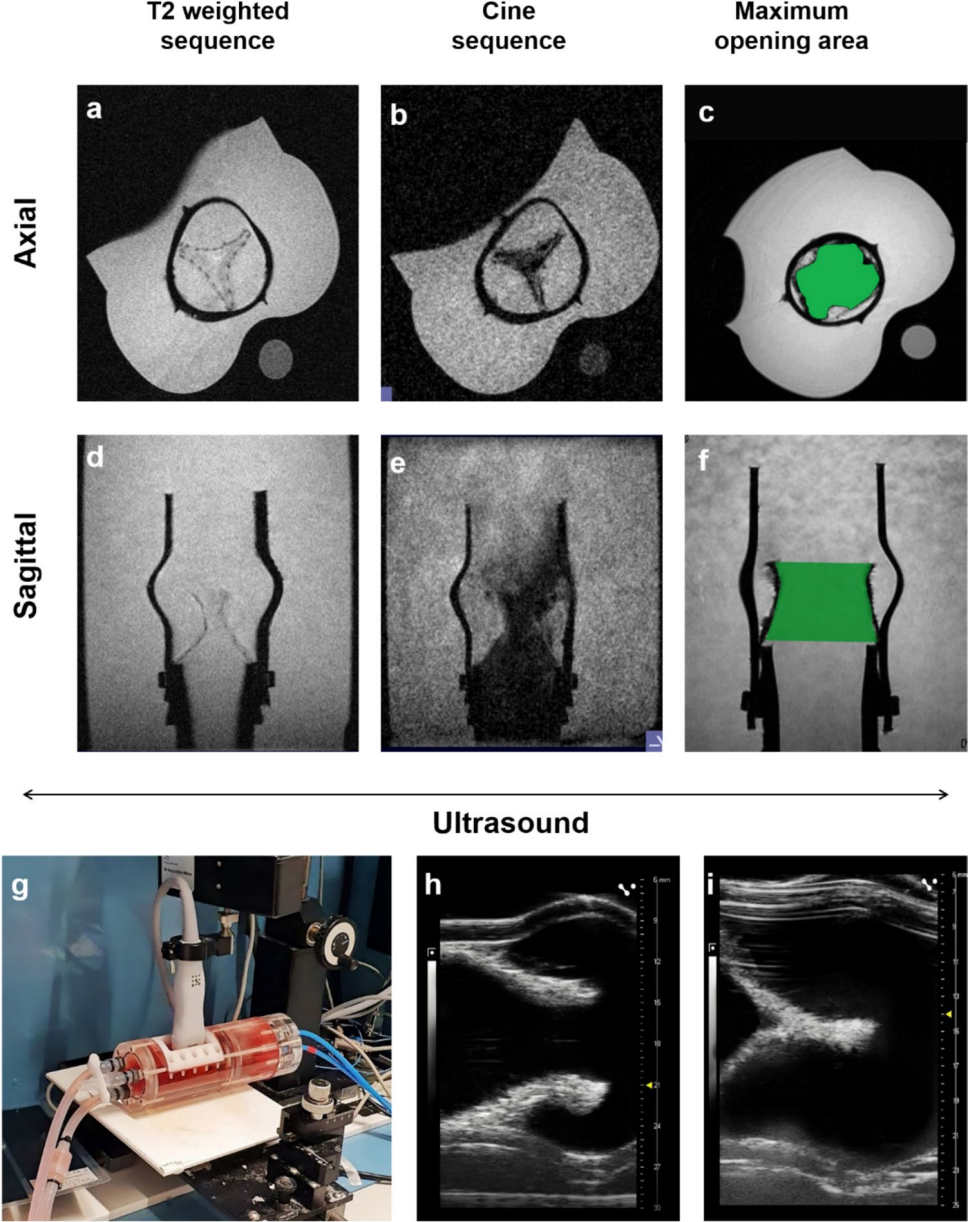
MR and ultrasound imaging of the TEHVs. a–c Axial views of the TEHVs in both T2-weighted, cine sequence, and maximum opening area. d–f Sagittal view of the TEHVs in both T2-weighted, cine sequence, and maximum opening area. g The bioreactor setup is on the ultrasound bench where the transducer is on a movable axis. h Opening frame of the TEHVs. i Closing frame of the TEHVs
